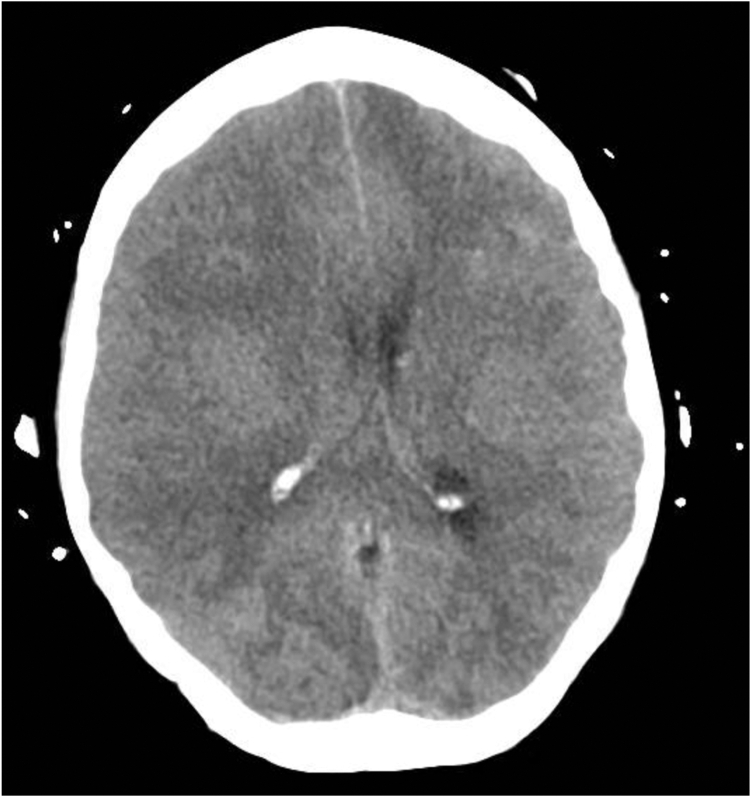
Brain computed tomography demonstrating severe diffuse anoxic brain injury, cerebral edema, midline shift, and transtentorial herniation.
